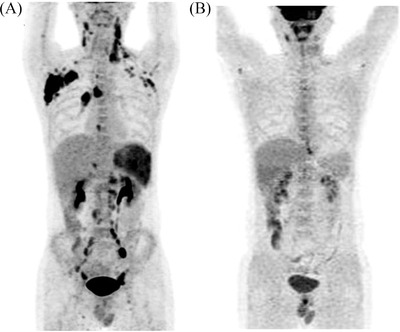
Comparison of curative effect of patients before (A) and after (B) progression disease programmed death‐1 (PD‐1) antibody alone treatment through positron emission tomography (PET)/computed tomography (CT) image examination
